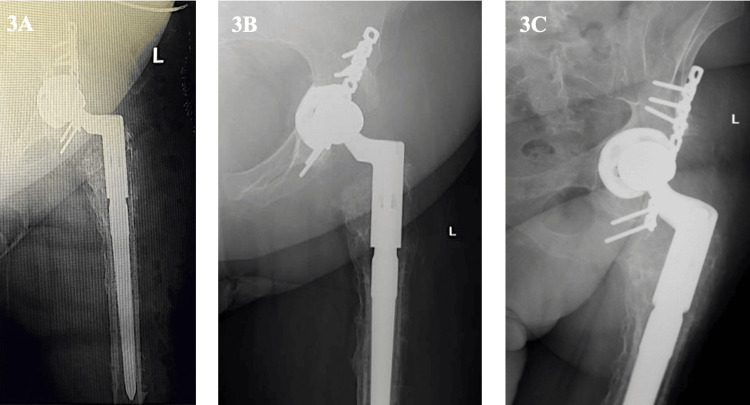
Postoperative plain radiographs of the left hip joint Postoperative plain radiographs of the left hip joint post conversion to total hip arthroplasty
